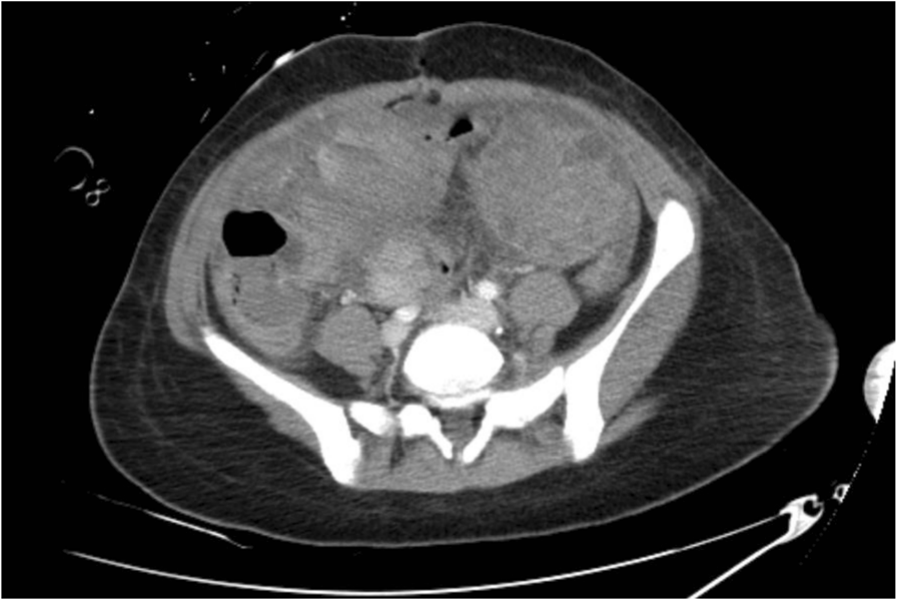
Image of the ovaries of the patient from the computed tomography scan
